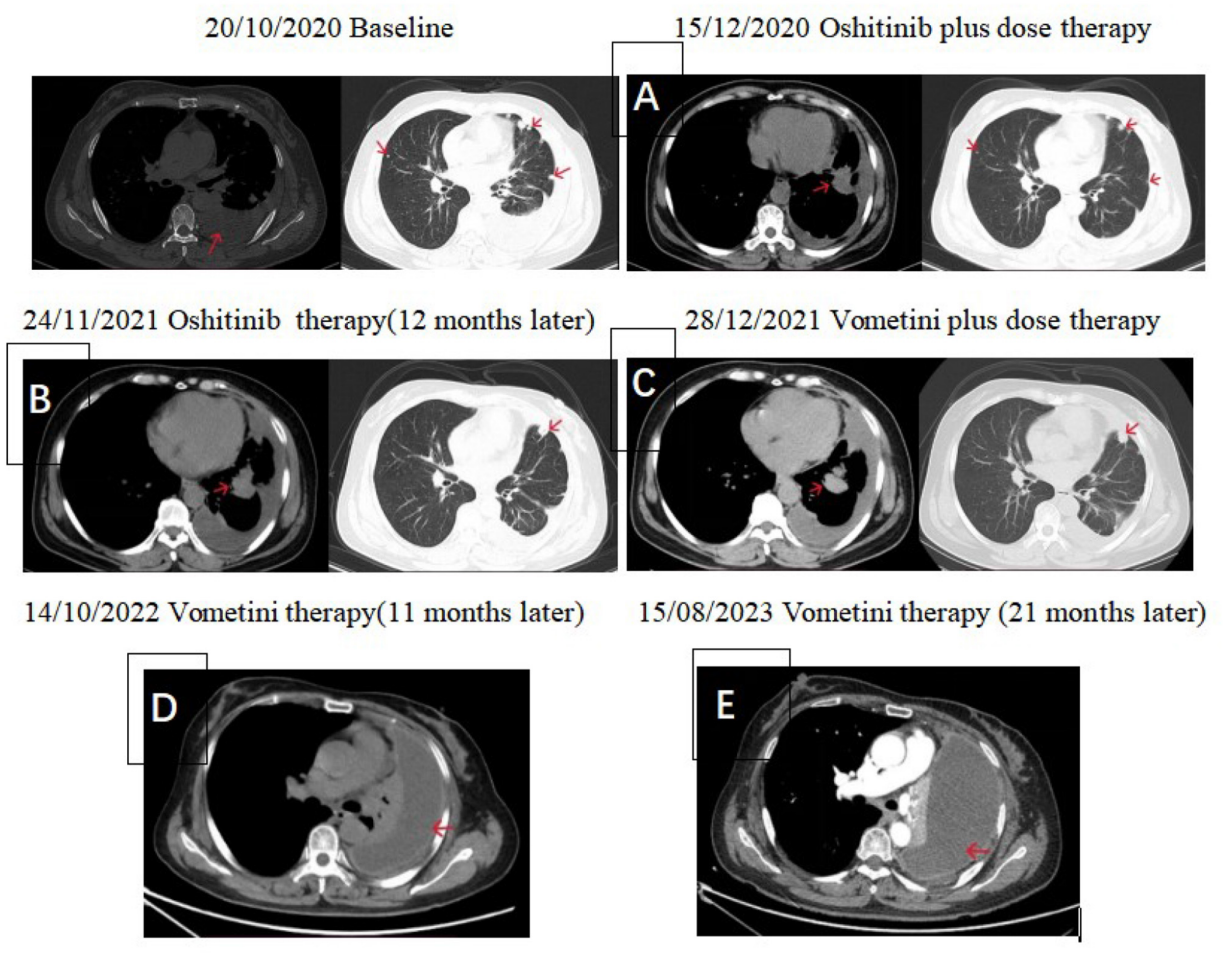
Lung computed tomography images from right to left, respectively, from October 2020 to August 2023. (A) After receiving an increased dose of osimertinib, the patient's condition remained stable, the pleural effusion was significantly reduced, the left lung lesions were exposed, and the metastatic lesions in both lungs were reduced. (B) After 12 months of increased-dose osimertinib, the primary and metastatic lesions in the left lung were significantly enlarged. (C) Following dose escalation of furmonertinib, the lung lesions were largely stable. (D) After 11 months, the patient developed a left pleural effusion. (E) At 21 months, follow-up revealed recurrence of pleural effusion.
